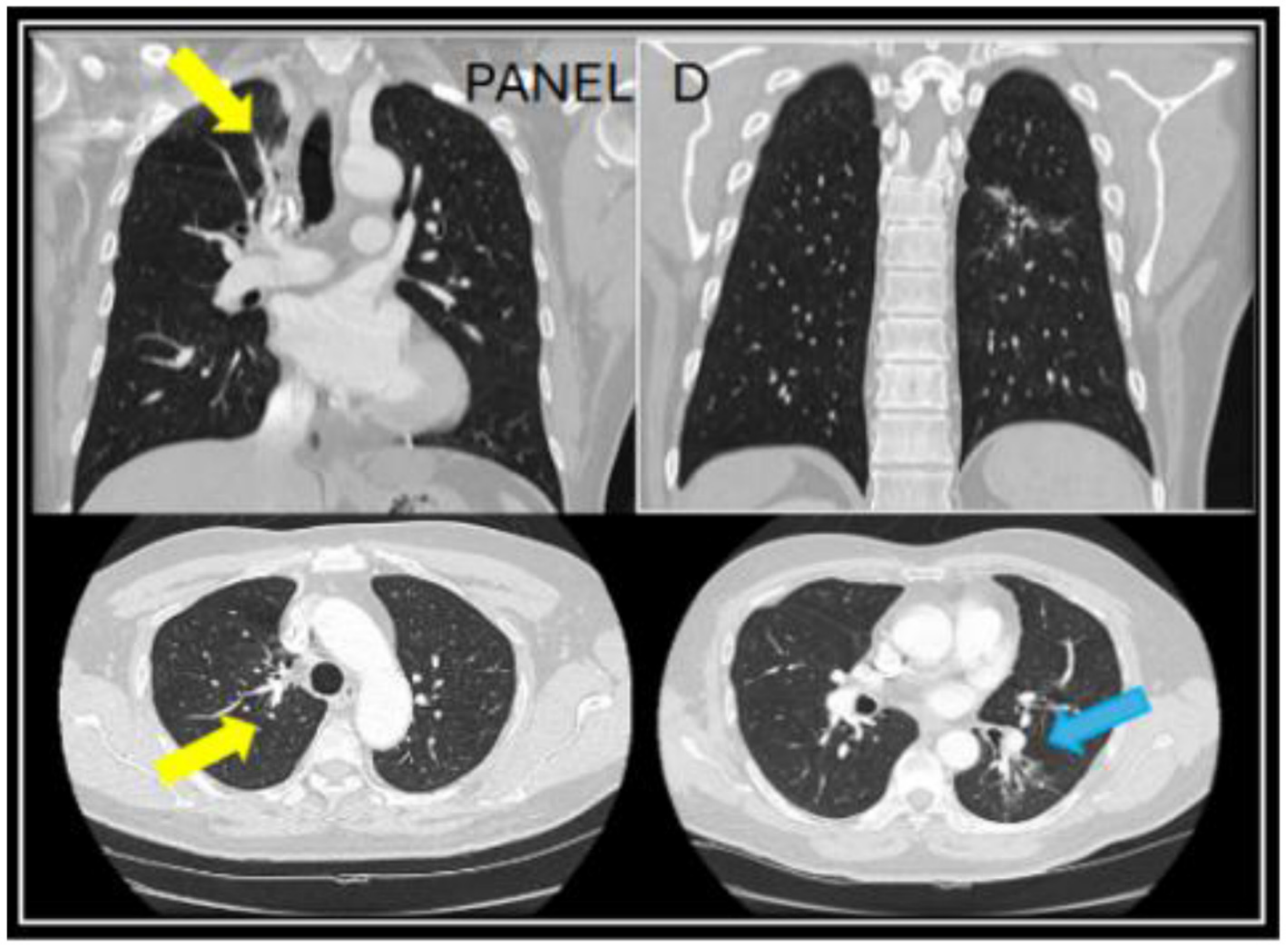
A follow up CT chest done at year four while being on long-term itraconazole showed resolution of the right upper lung infiltrate (yellow arrows) and residual reticulated bronchiectasis in the left lower lobe [blue arrow in (D)].
